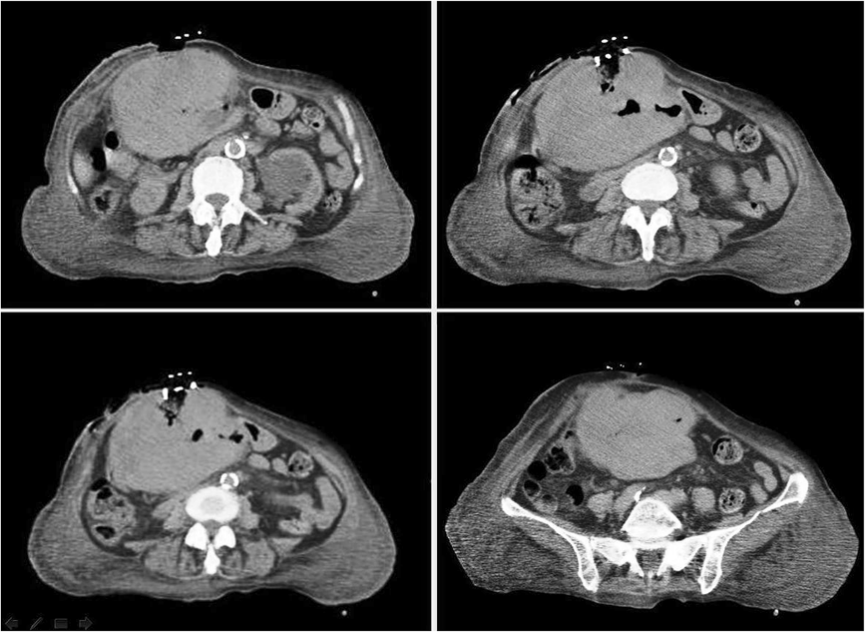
Computed tomography scan: huge mass arising from the gastric antrum, infiltrating and perforating the abdominal wall.
